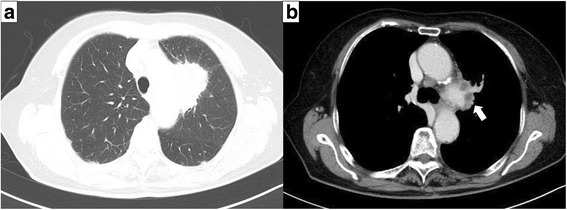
Preoperative chest computed tomography (CT) scan. a Chest CT scan showing a 5 cm tumor in the *left upper lobe*. b Tumor was suspected to invade the pulmonary artery (*arrow*)
